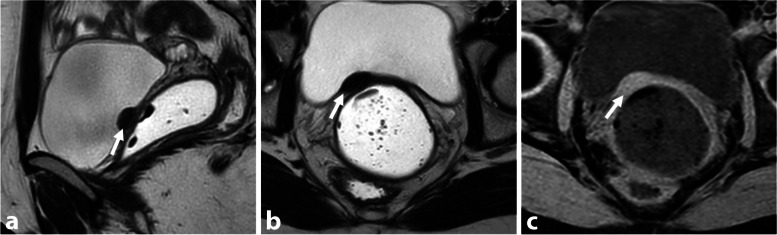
Unilateral right-sided vesicoureteral reflux surgical bulking agent (Macroplastique®—polydimethylsiloxane injection) in a 26-year-old woman. a Sagittal and (b) axial T2-W MR images show a T2-hypointense unilateral right-sided vesicoureteral reflux implant (arrows) at the ureterovesical junction. c Axial T1-W fat-suppressed MR image shows an ovoid geometrical shape in T1-isosignal intensity (arrow)
